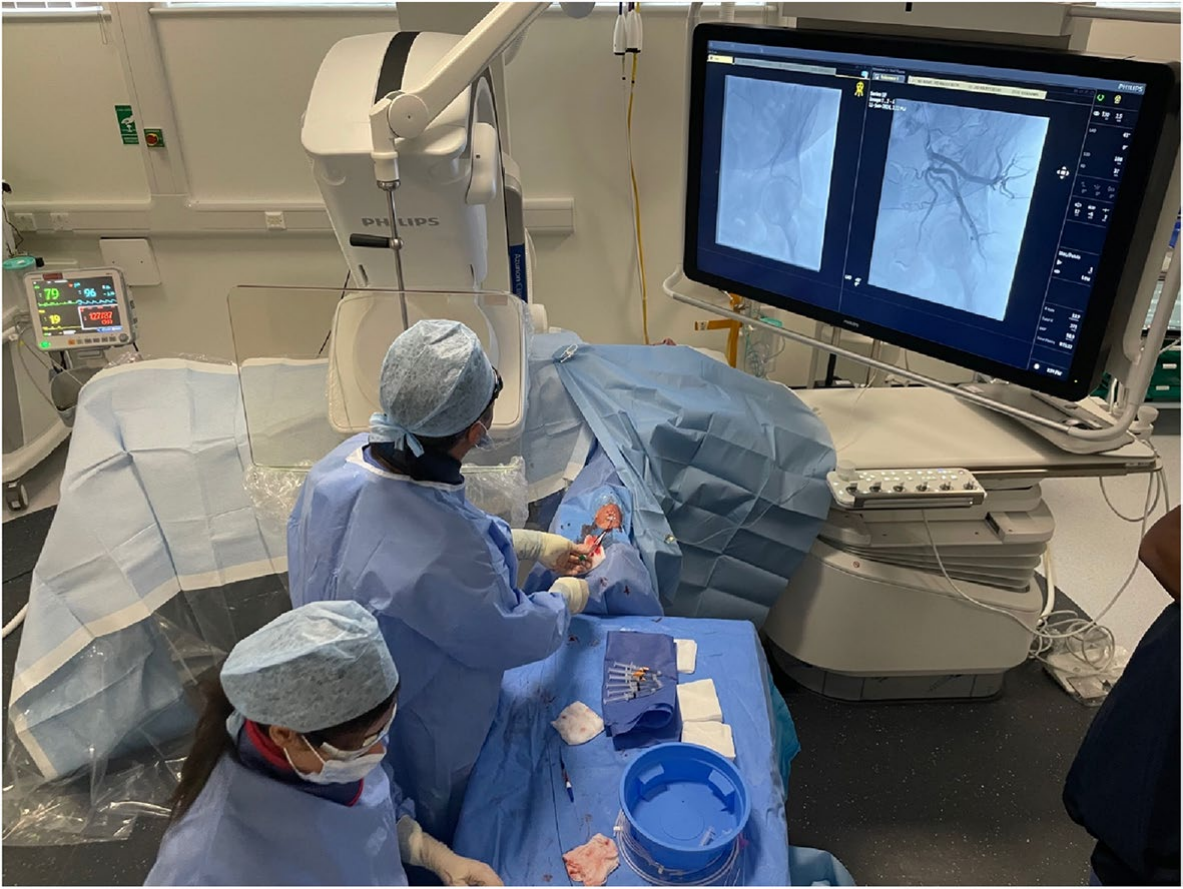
Room setup for PAE. The left arm is abducted on an arm board. The figure shows the positions of the operator, nurse, and technician, as well as the optimal placement of radiation protection and the angiographic machine (cone beam CT)
